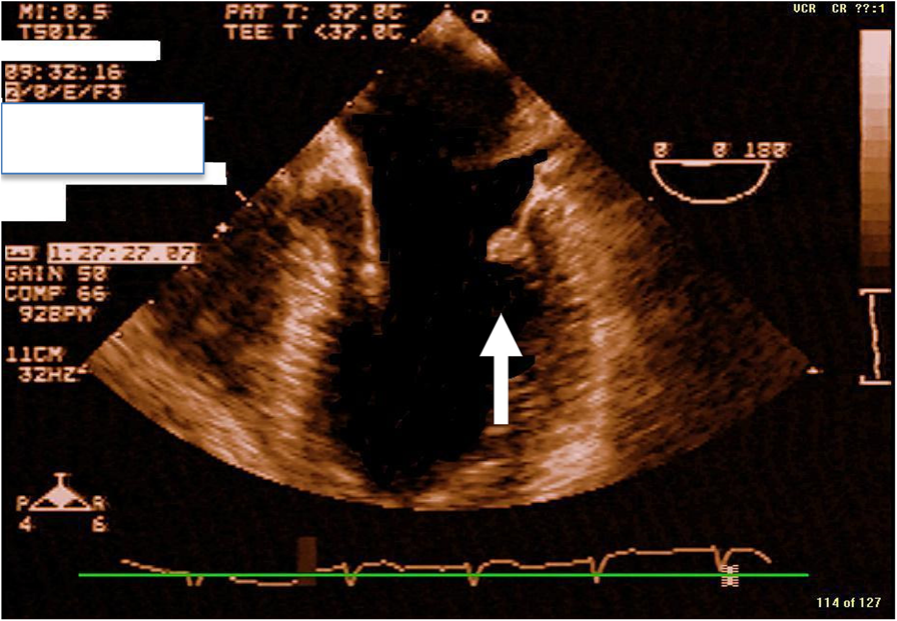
Transesophageal echocardiogram depicting mitral valve mass
